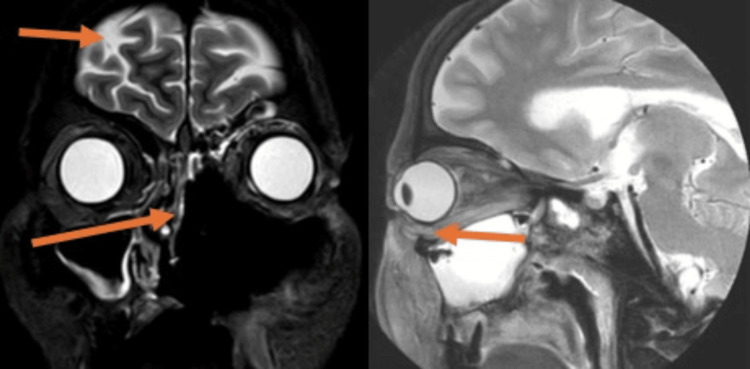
MRI coronal section STIR sequence and sagittal T2W FS sequence. The orange arrows depict meningeal involvement, and maxillary sinus with middle and inferior turbinate extension along with the protruded left globe and a bulky extraocular muscle STIR: short tau inversion recovery; T2WI: T2-weighted image; T2W FS: T2-weighted fat saturation
